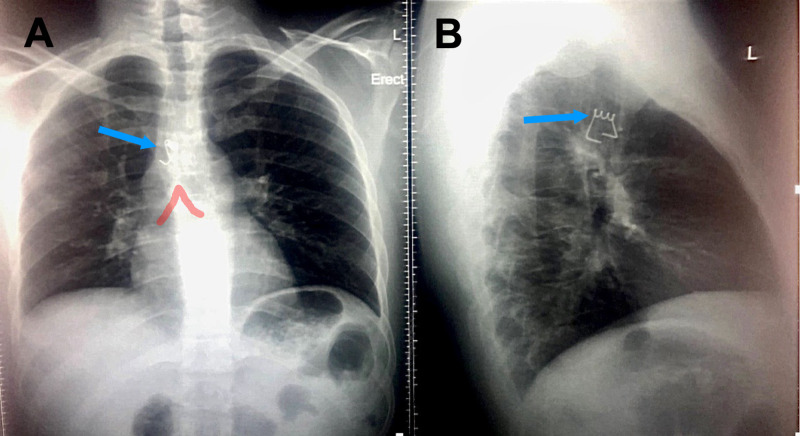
Chest radiograph: anterior-posterior (A) and lateral (B) views. (A) demonstrates the radio-opaque metal spring (blue arrow) just above the carina (red line) at the level of the right main bronchus. (B) confirms the location of the object (blue arrow) in the trachea, situated anterior to the esophagus.
